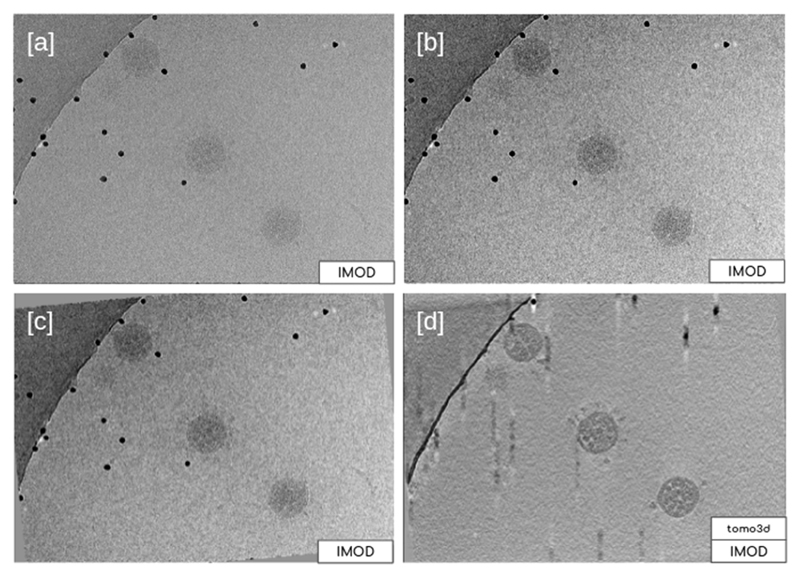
Results obtained for some of the steps carried out to reconstruct TS_293 of dataset EMPIAR-10453 [a] and [b] correspond to the original tilt series and preprocessed tilt series, respectively, being [b] the result of the tilt series X-ray erasing dose filtering, and downsampling to bin 4. Sub-figure [c] is the result of the automatic fiducial alignment offered by plugin scipion-em-imod, and sub-figure [d] is the result of the SIRT reconstruction performed with plugin scipion-em-tomo3d without CTF estimation in this case (it corresponds to the green box named “Imod - Tomo reconstruction (wbp)” in Fig. 7). All the subfigures represent data displayed with viewer IMOD-3dmod.
